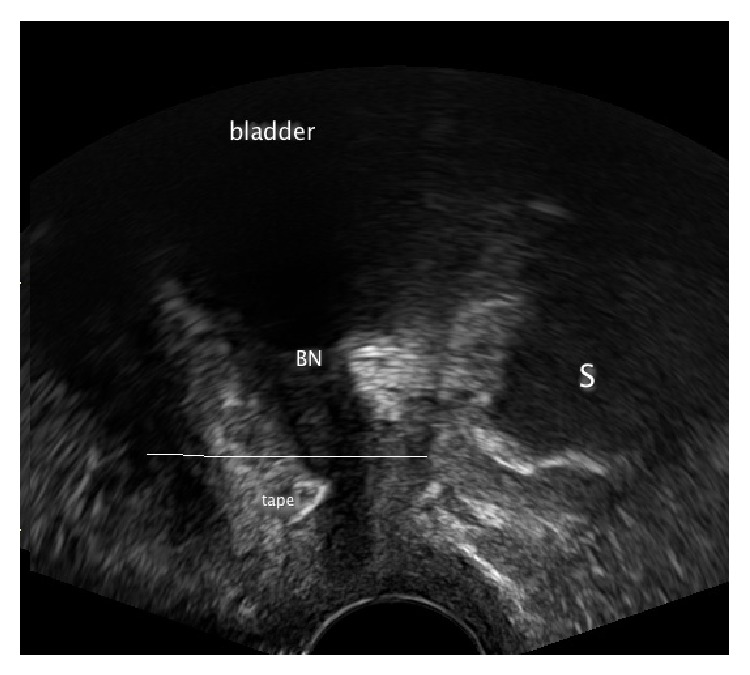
Pelvic floor ultrasound examination in sagittal plane. S: symphysis pubis and BN: bladder neck. The sling lies below the middle part of the urethra.
